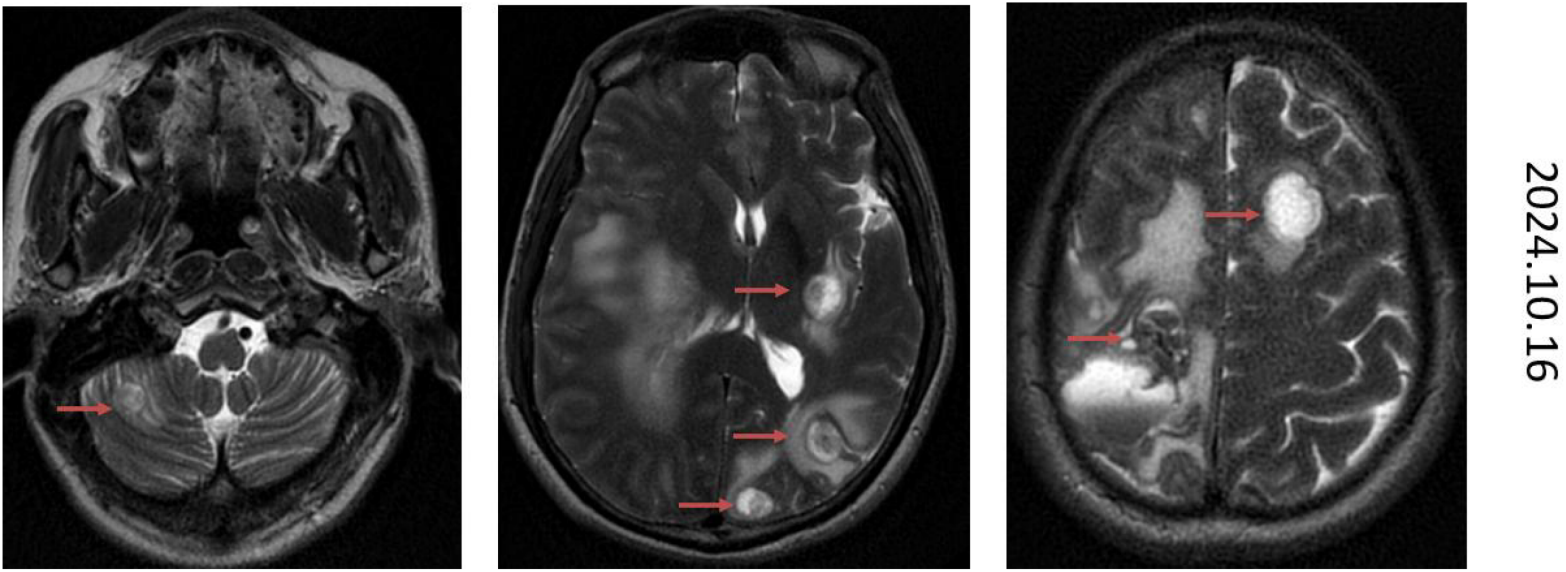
October 16, 2024 contrast-enhanced magnetic resonance imaging (MRI) of the brain demonstrates characteristic radiographic features consistent with cerebral metastases.
